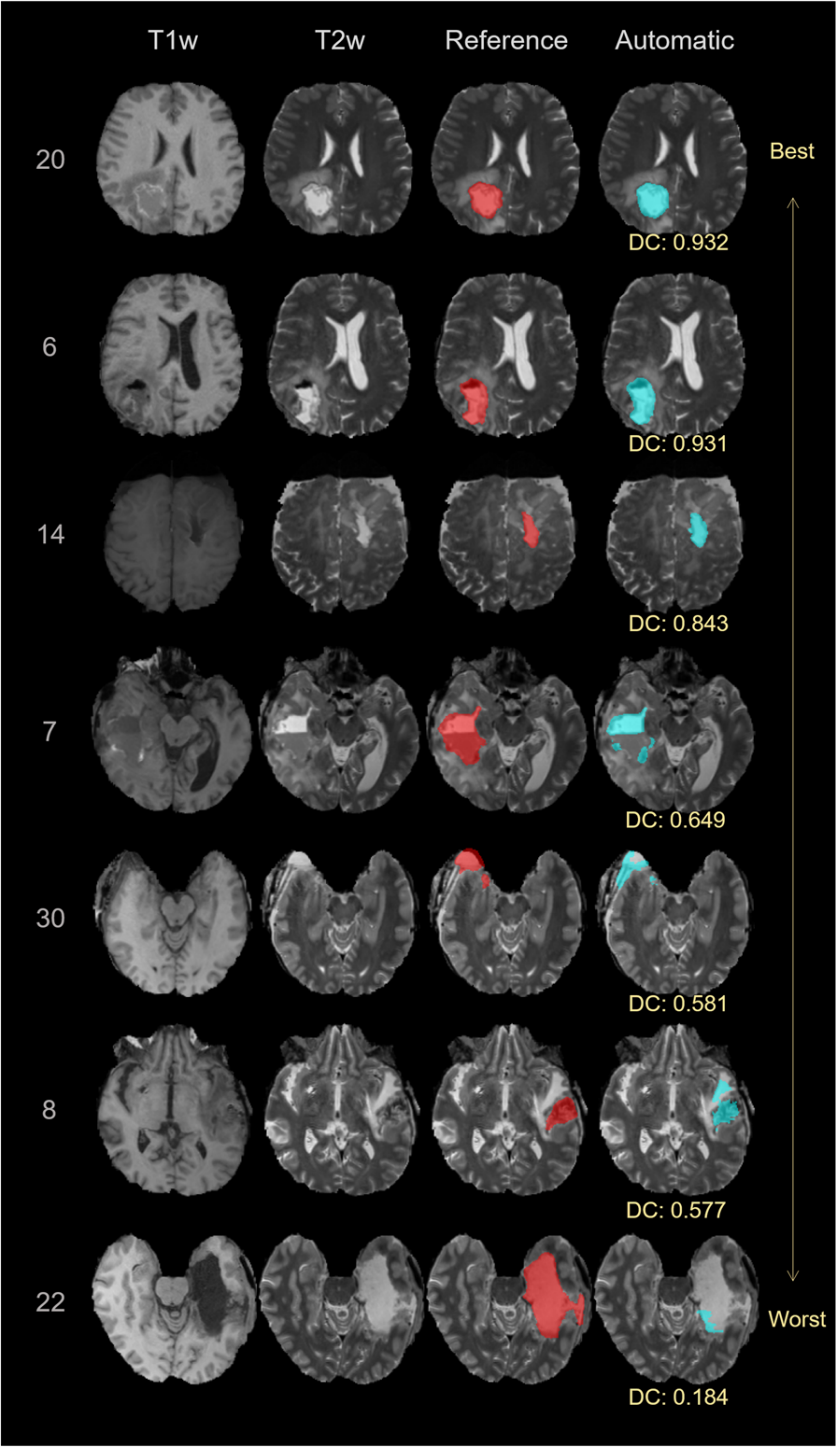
Representative axial slices of the produced segmentations in comparison to the expert consensus. The rows correspond to different cases and are listed according to the segmentation performance in terms of Dice coefficient (DC). The columns show the T1-weighted (T1w) image, the T2-weighted (T2w) image, the expert consensus (reference) and the automatic segmentation (as overlay on the T2w)
